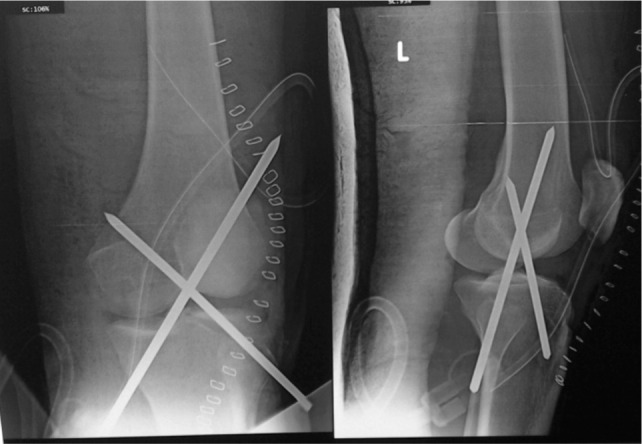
Antero-posterior and lateral view of radiographs showing reduction of joint and fixation with two crossed Steinman pins
